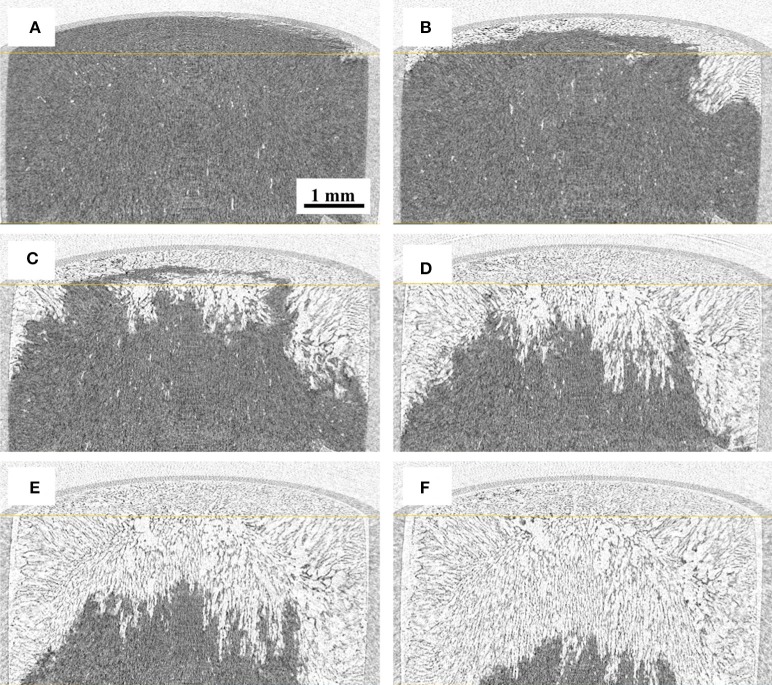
3D X-ray CT images during freeze-drying of the samples annealed for 12 h, dried for (A) 600, (B) 1200, (C) 1,800, (D) 2,400, (E) 3,000, and (F) 3,900 s.
